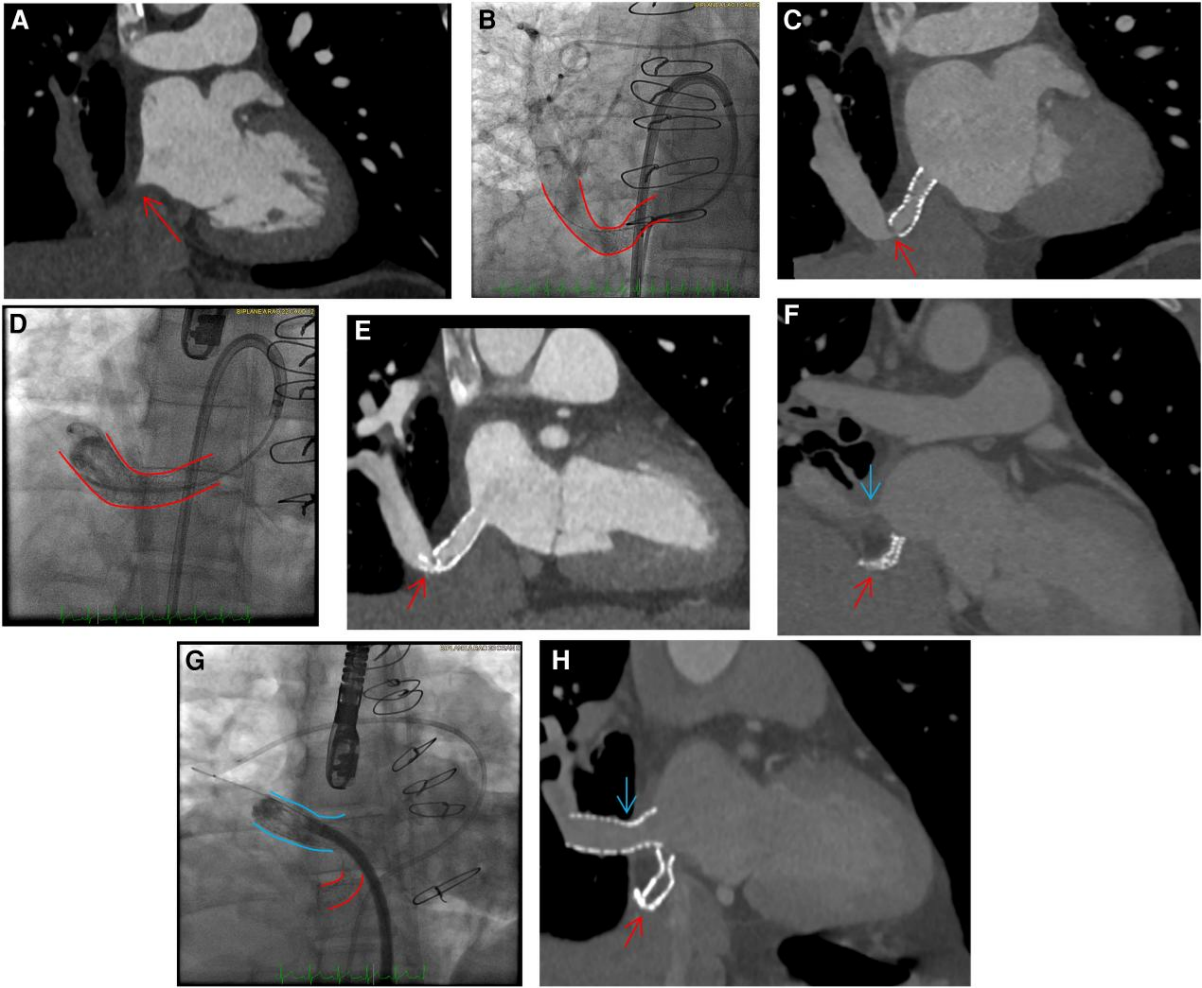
Case 2. (*A*) Computed tomography scan at 8 months post-surgery showing obstructed baffle (arrow). (*B*) Fluoroscopy showing patent baffle following intervention (solid line indicating baffle). (*C*) Computed tomography scan at 12 months post-stenting showing kinking of the vein (arrow). (*D*) Fluoroscopy from second intervention showing patent baffle after second stent (solid lines indicating baffle). € Computed tomography scan at 11 months after second stenting procedure showing crushed stent (arrow). (*F*) Computed tomography scan at 6 months after re-do surgery with pericardial roll showing obstructed original baffle (lower arrow) and obstructed new pericardial roll (upper arrow). (*G*) Fluoroscopy showing first obstructed baffle (lower solid lines) and now patent pericardial roll post-stenting (upper solid lines). (*H*) Computed tomography scan at 6 months after intervention showing patent-stented pericardial roll baffle (upper arrow) and obstructed original baffle (lower arrow).
